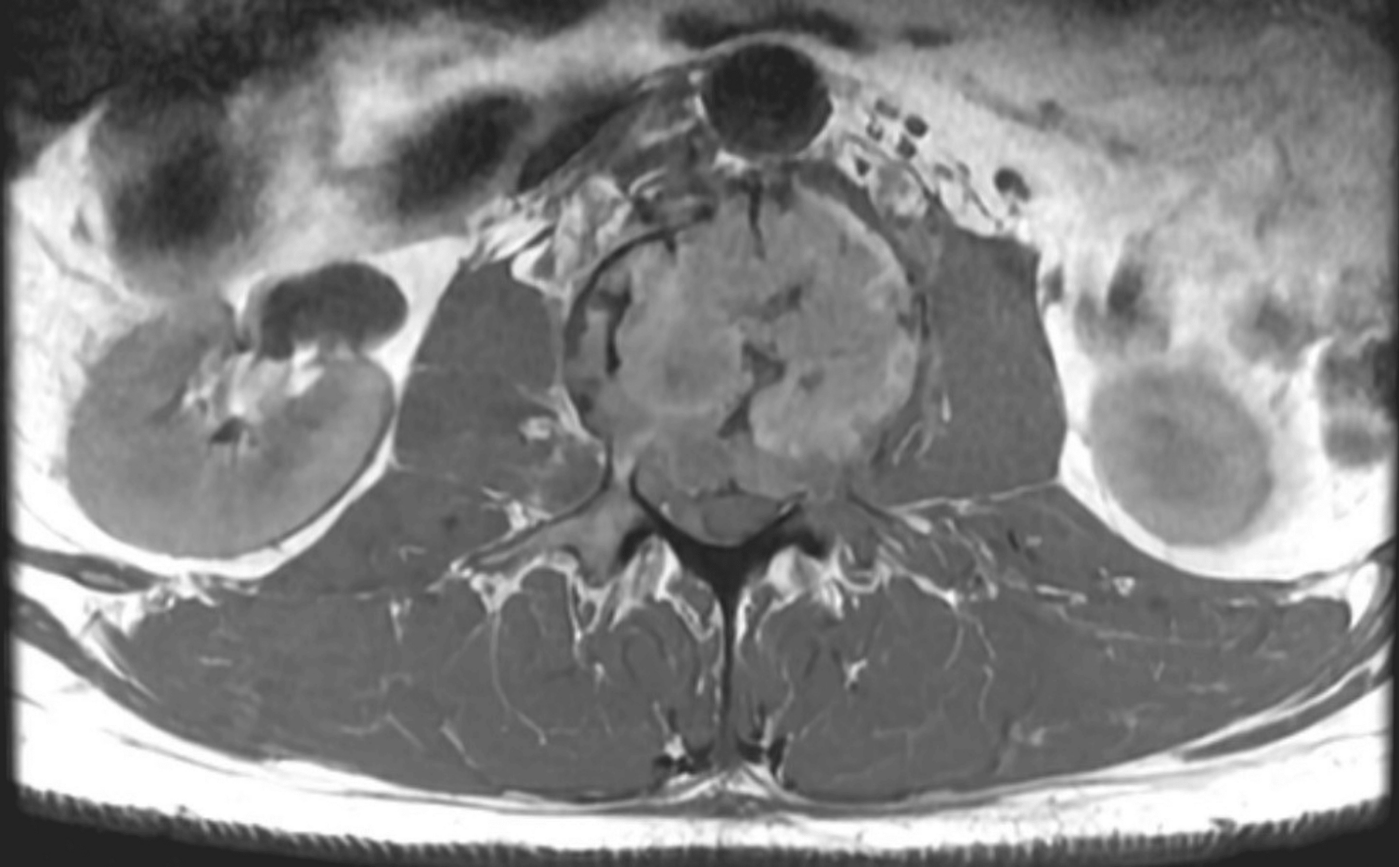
Axial section of MRI at the level of L2 vertebrae showing destructive changes of L2 vertebrae MRI: magnetic resonance imaging, L2: second lumbar vertebrae
